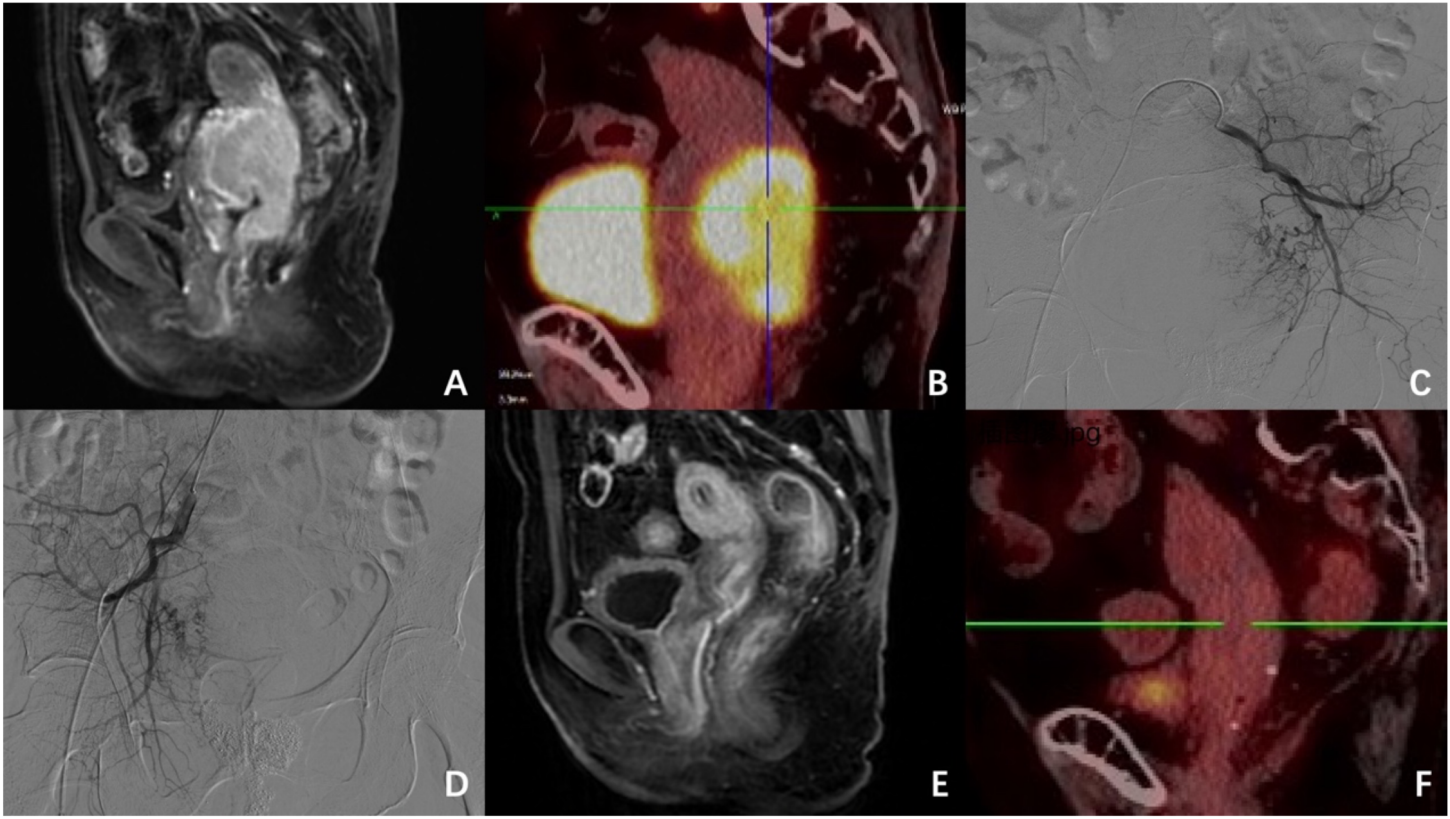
Imaging and DSA findings of Case 3: (A) Pelvic MRI demonstrates a 6.5×4.5 cm heterogeneously enhancing cervical mass with posterior extension to the rectal serosa. (B) PET-CT reveals markedly increased metabolic activity (SUVmax 14.2) in the cervical lesion. (C, D) Bilateral uterine artery angiography shows hypervascular tumor supply with intense neovascularization. (E, F) Follow-up MRI and PET-CT at 1-month post-combination therapy confirm complete resolution of the primary lesion without residual metabolic activity.
